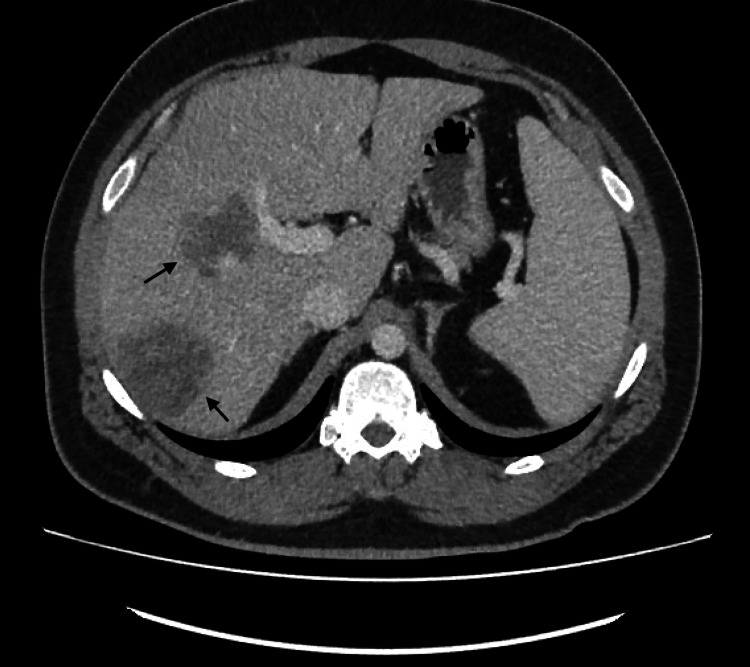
CT abdomen CT abdomen showing lesions in the middle (arrow above) and inferior right lobe (arrow below) of the liver
